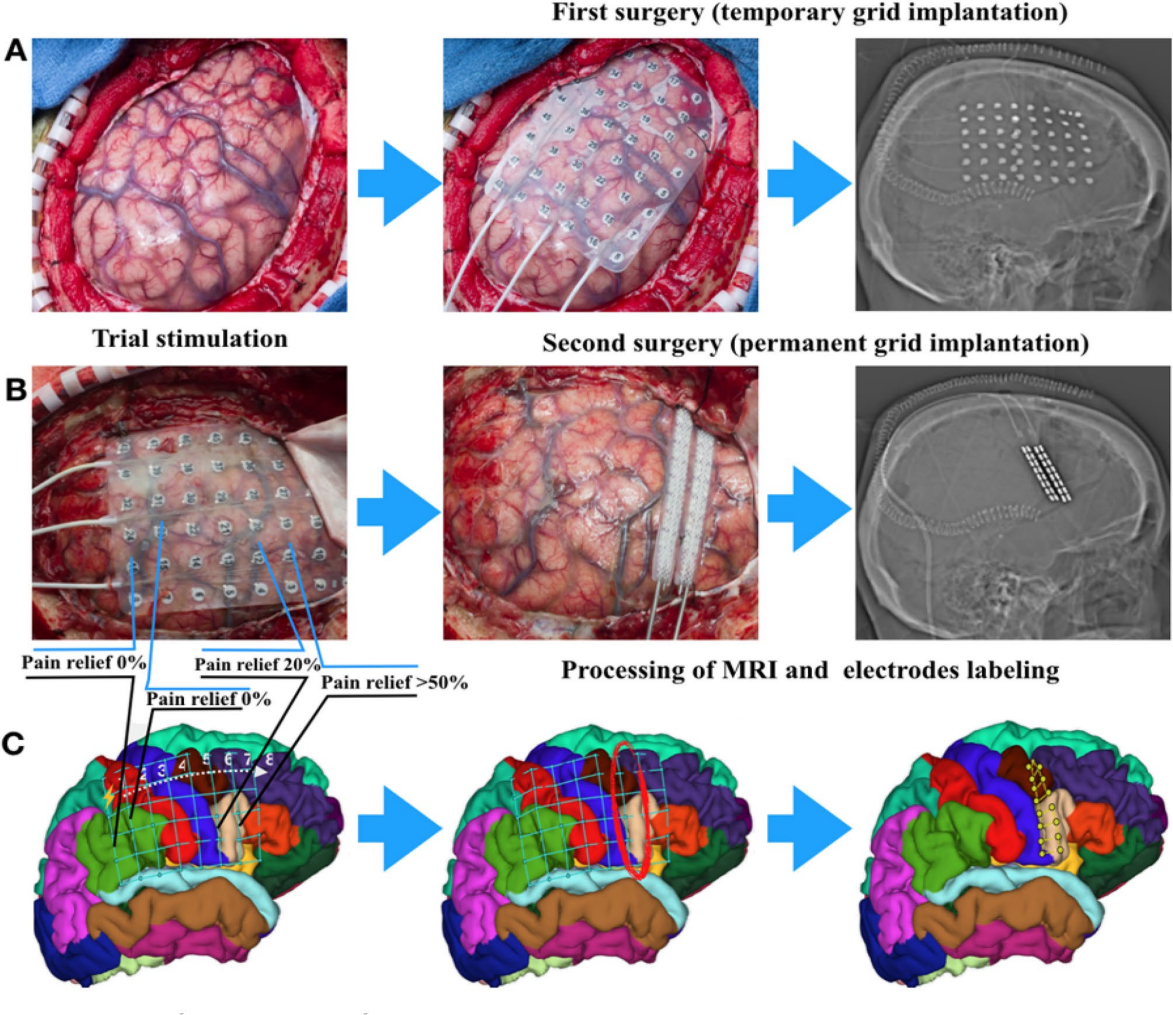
Surgical intervention and mapping. (A) Surgery for temporary grid placement in order: craniotomy; 6 × 8 temporary grid placement; X-ray/CT-scan representative for temporary grid position. (B) Trial stimulation for target optimization, with following permanent grid implantation over identified area. X-ray/CT-scan representative for permanent grid position. (C) Further image processing to reveal electrodes position over exact cortex area according to Desican-Killiany atlas.
